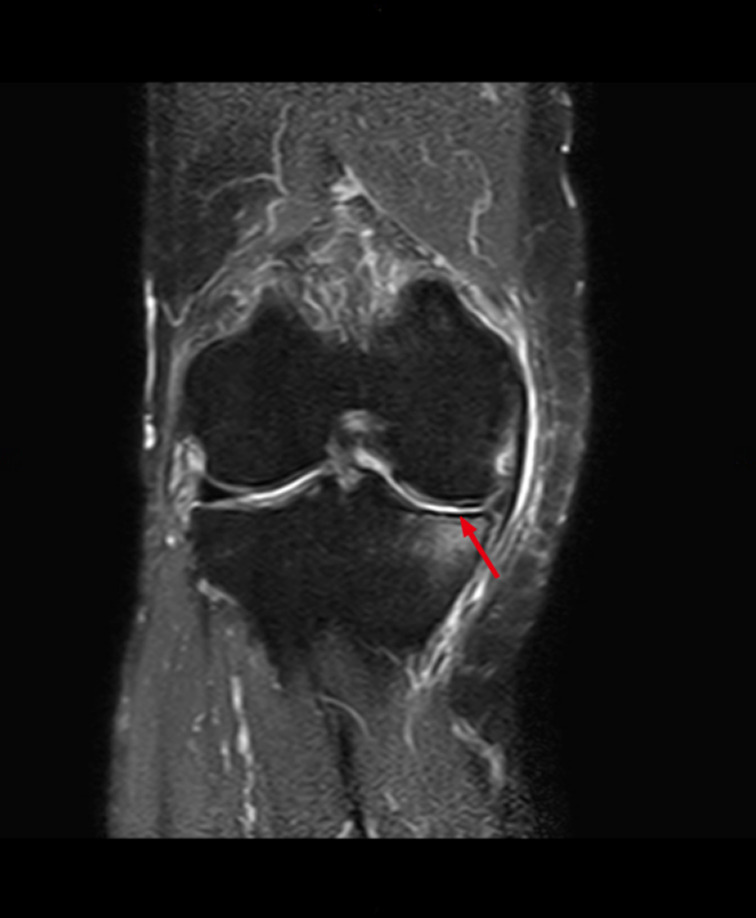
Measurement of the severity of KOA. Coronal intermediate‐weighted fat suppressed MRI shows focal cartilage damage (red arrow).
